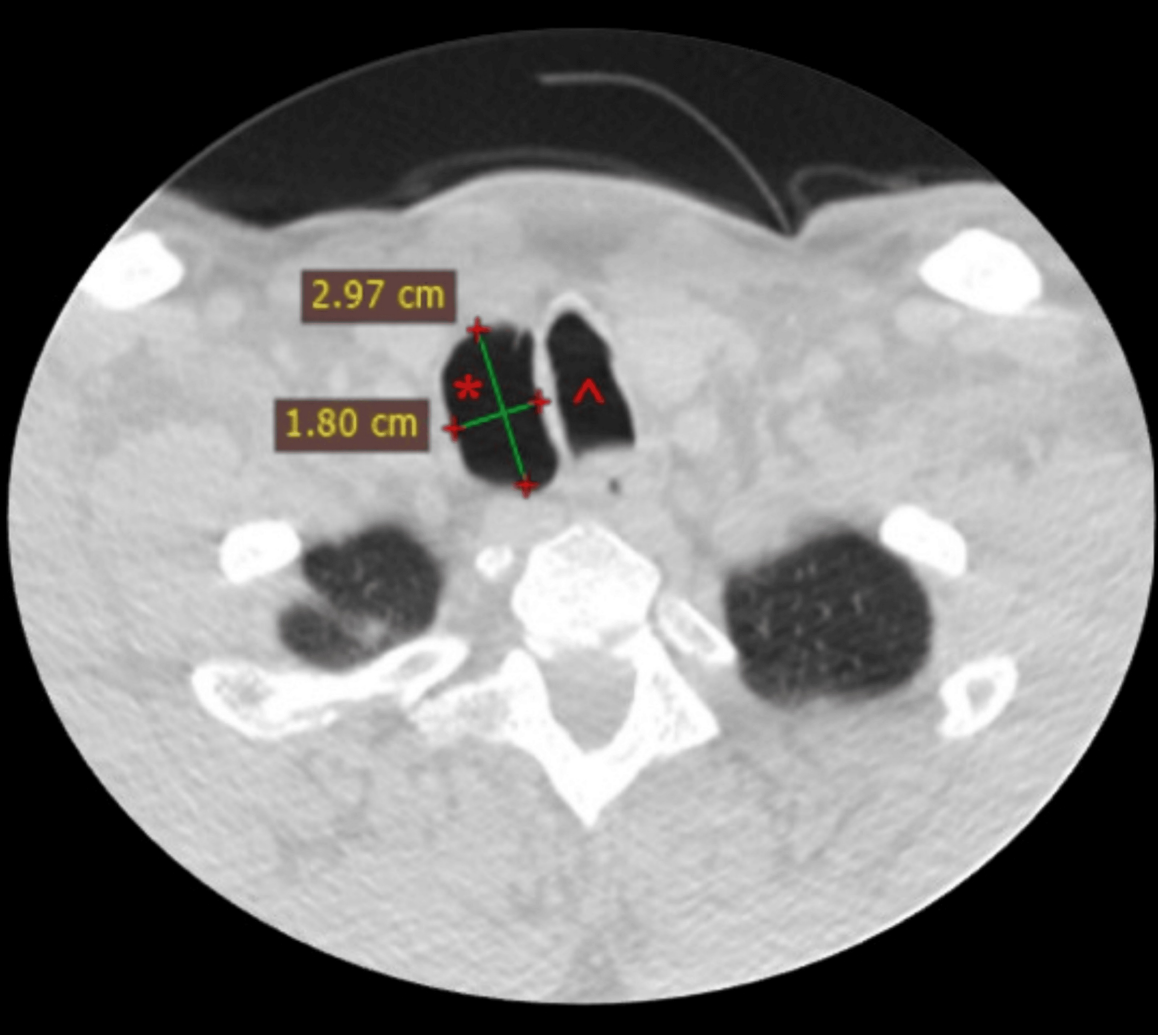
Thoracic CT scan (1.25-mm slice), axial view Right-sided tracheal diverticulum (*) with dimensions of 2.97 x 1.80 cm, without detectable connection to the trachea (^)
